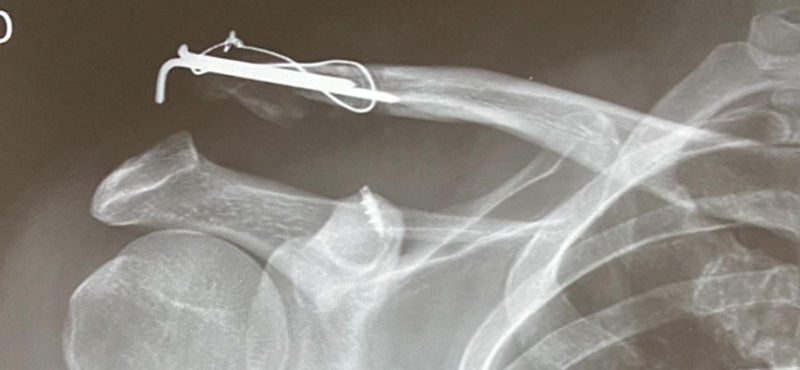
Radiograph revealing an increased coracoclavicular space due to rupture of the anchor wires and consequent new acromioclavicular dislocation.
